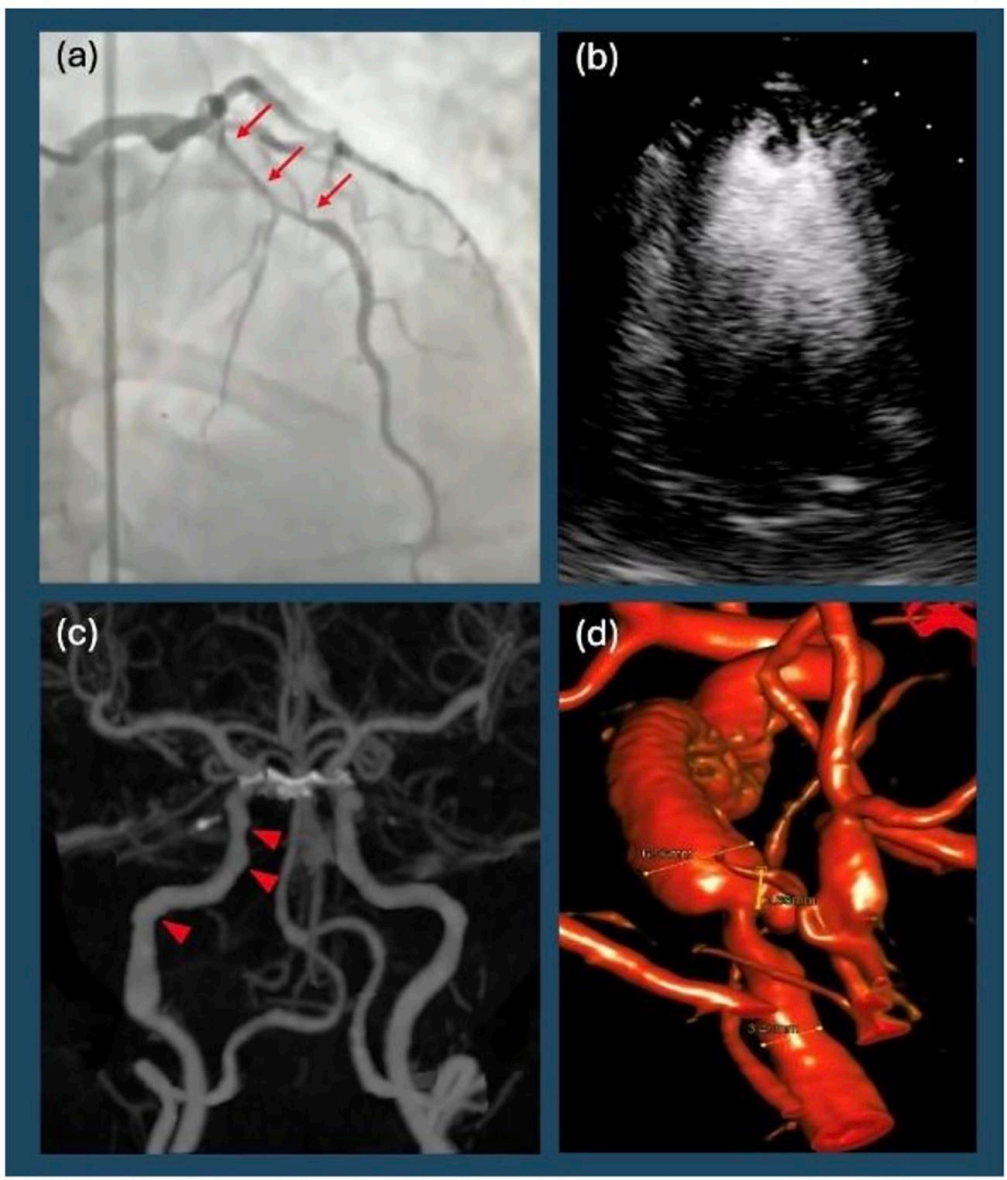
Diagnostic imaging. (a) Patient's initial left heart catheterization showing diffuse, long, smooth stenosis of the mid left anterior descending artery (arrows), consistent with Type II spontaneous coronary artery dissection. (b) Transthoracic echocardiogram with contrast showing apical left ventricular thrombus in addition to reduced ejection fraction. (c) Computer Tomographic angiography of the head and neck revealing focal beaded irregularity of right internal carotid artery (arrowheads) consistent with fibromuscular dysplasia. (d) Digital subtraction angiography showing right distal internal carotid artery dissection with partially thrombosed pseudoaneurysm.
